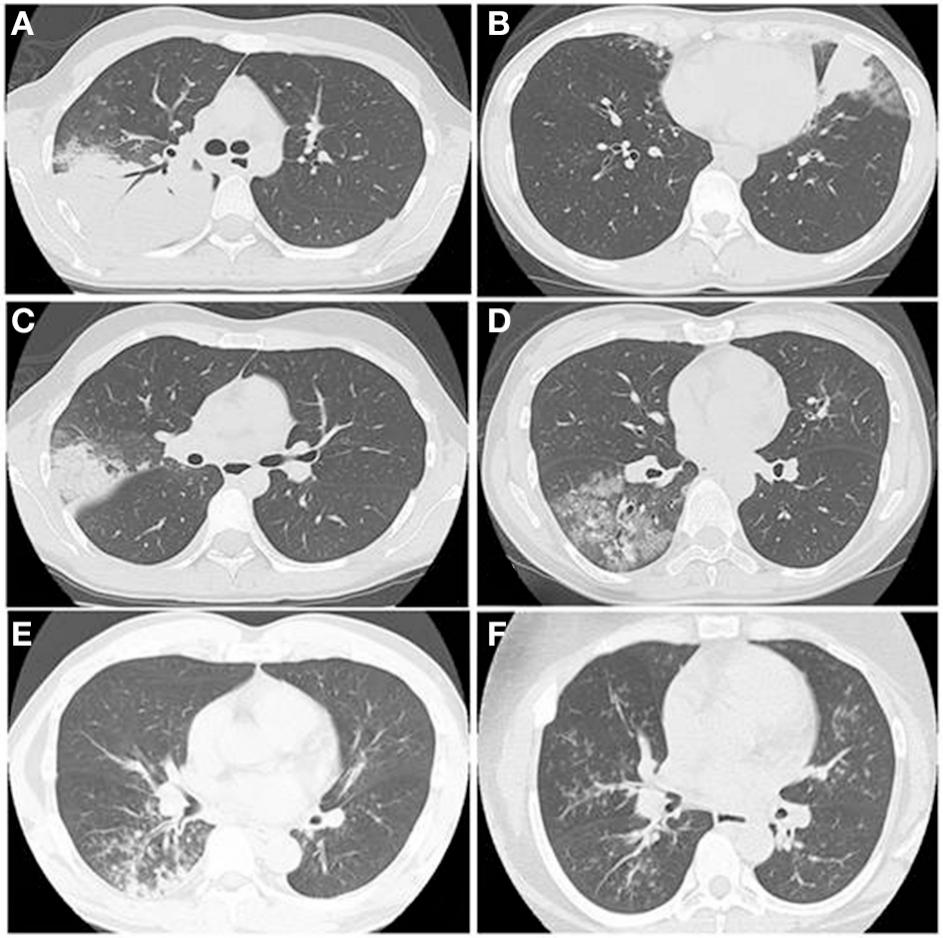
The HRCT findings of Mp pneumonia are characterized as (A) consolidation with air bronchogram surrounded by a crazy paving appearance, (B) consolidation with reticular shadow, (C) consolidation with GGO, (D) GGO with interlobular septal thickening, crazy paving appearance, (E) bronchial wall thickening with centrilobular nodules, (F) diffuse centrilobular nodules.
